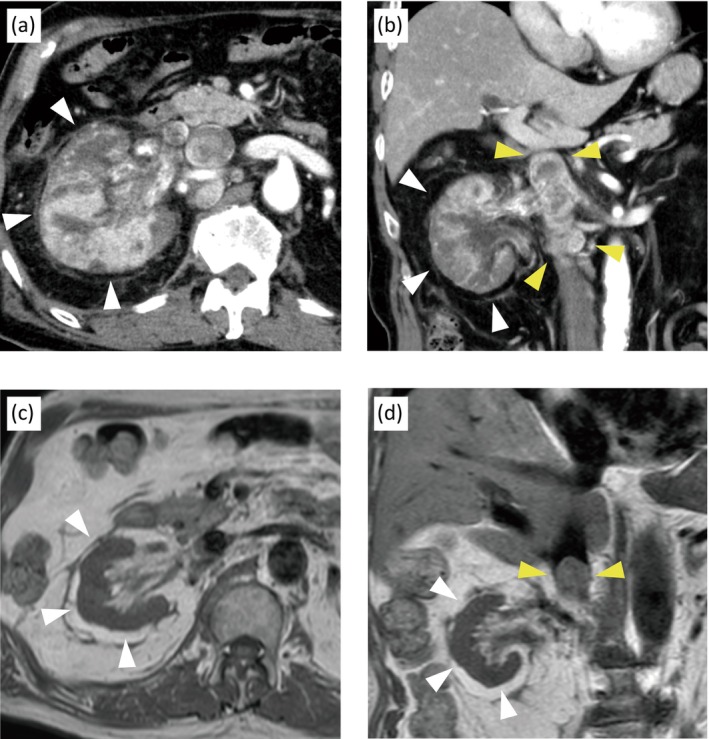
Radiological findings before and after systemic therapy. (a) Axial and (b) coronal contrast‐enhanced computed tomography scans showing a right renal mass with extension into the inferior vena cava (IVC) before systemic treatment. (c) Axial and (d) coronal T_1_‐weighted magnetic resonance imaging obtained after combination therapy of lenvatinib with pembrolizumab. White and yellow arrowheads denote the primary renal tumor and the IVC tumor thrombus, respectively.
